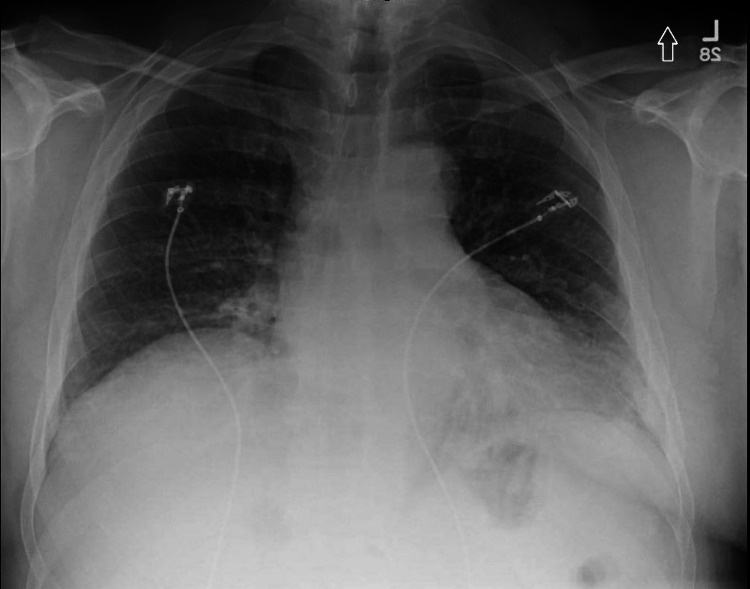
Chest X-ray showing mild left basilar atelectasis and/or infiltrate
